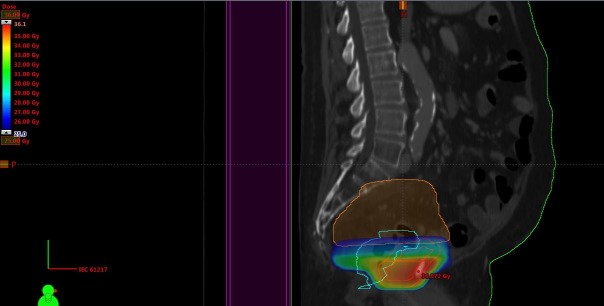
Sagittal computed tomography (CT) scan and dose distribution (course 3) Doses above 25 Gy are displayed, maximum dose 36 Gy.
